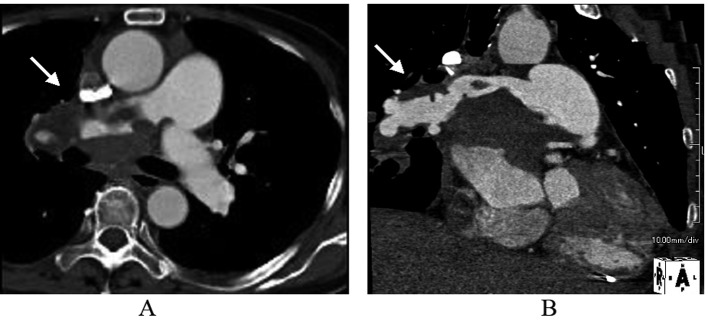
The image of Contrast-enhanced computed tomography scan. Early arterial phase showed massive filling defects in the proximal portion of right pulmonary artery and around the left atrium in axial (A) and sagittal (B) views.
